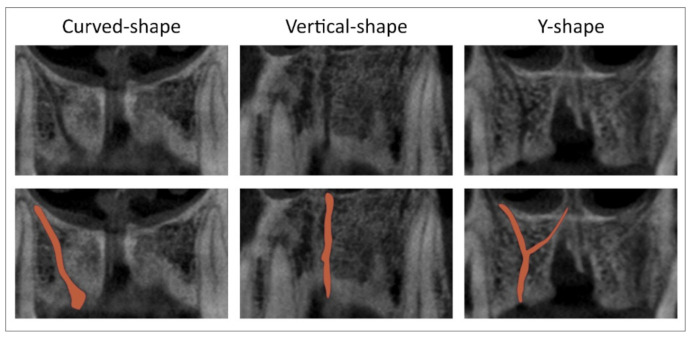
CBCT slices and marks of interest. Accessory canal shapes at the coronal cross-section (upper); red marks define ACs shapes at the coronal cross-section (below).
